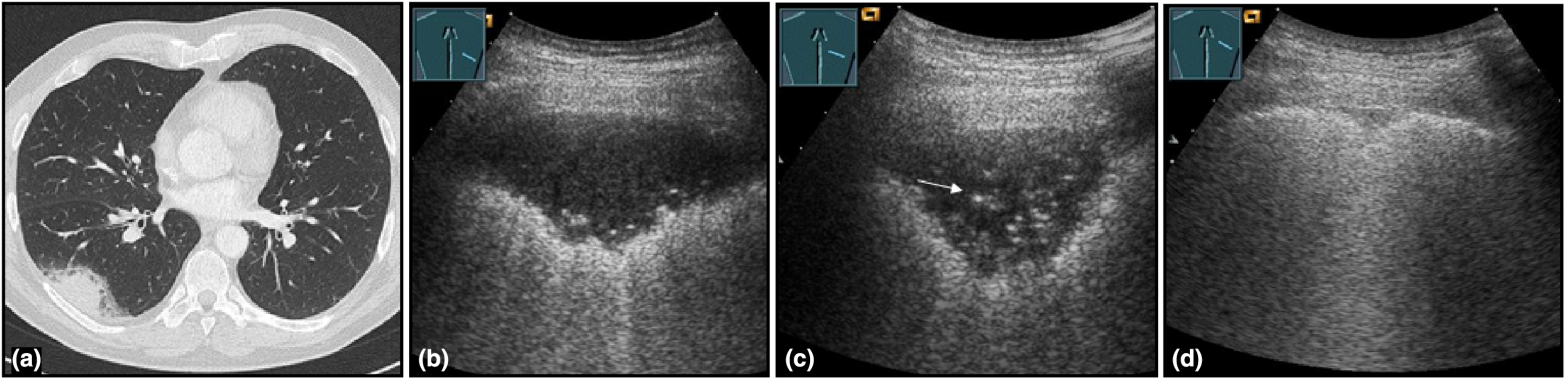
A 51‐year‐old patient presented to the emergency department with shortness of breath and respiratory pain. Computed tomography was performed to rule out pulmonary artery embolism. The computed tomography scan (courtesy of Prof. Dr. Andreas H. Mahnken, Department of Radiology, University Hospital Marburg) (a) shows a right‐sided dorsal consolidation; B‐mode ultrasound shows a homogeneous, hypoechoic consolidation (b), which regressed in size after 4 days with increasing air bronchogram (arrow) (c), and with almost complete regression after 4 weeks (d), compatible with community‐acquired pneumonia.
